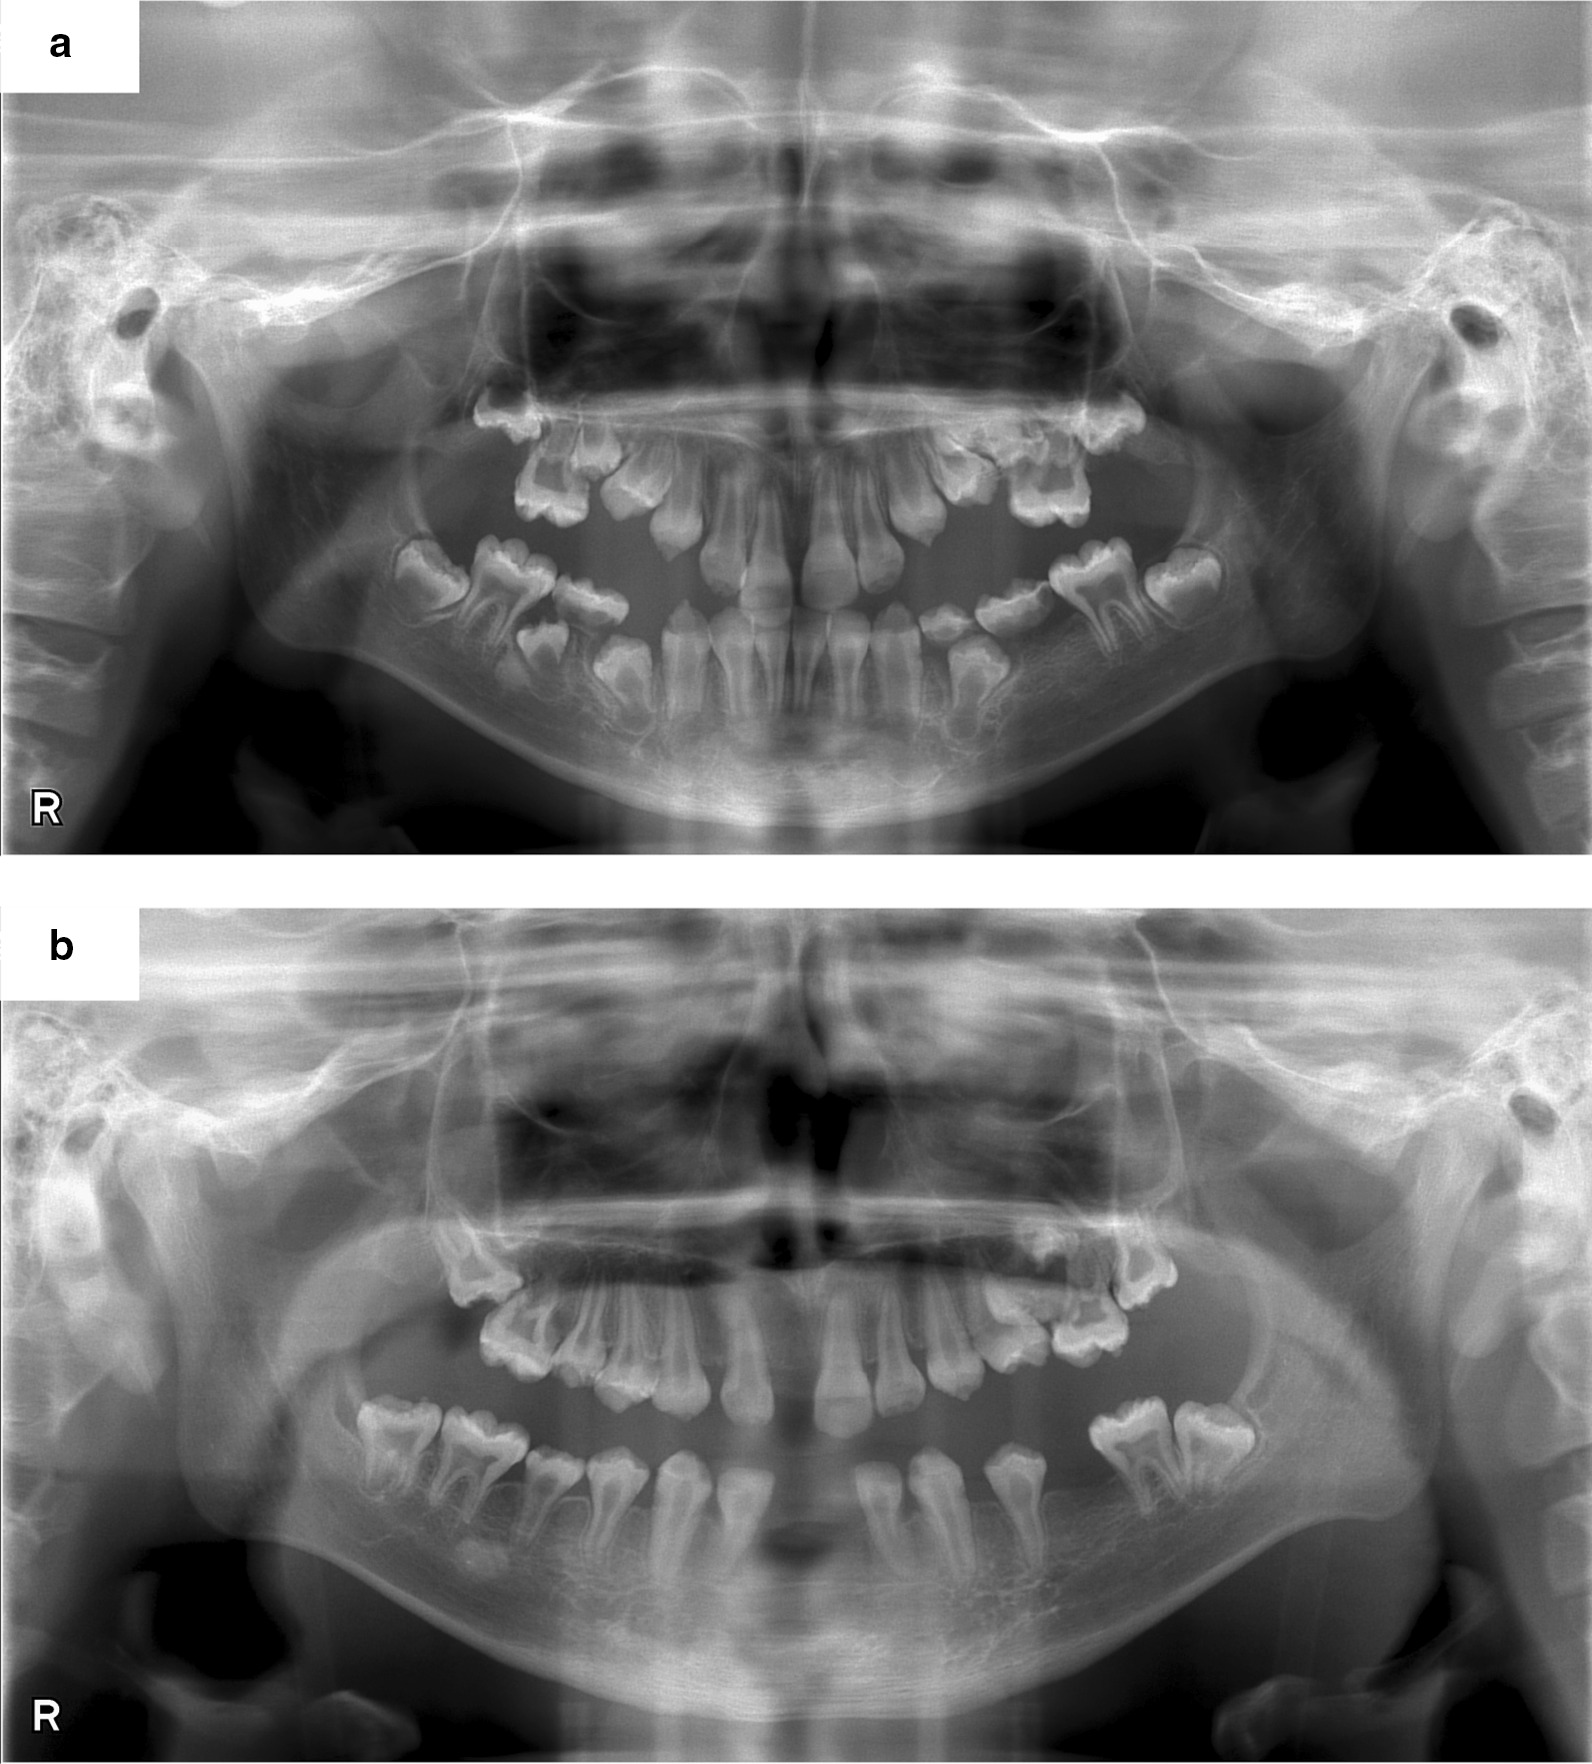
a Panoramic radiograph obtained at 11Y1M of age, prior to starting ERT. b. Panoramic radiograph obtained after starting ERT (patient age 14Y1M)
